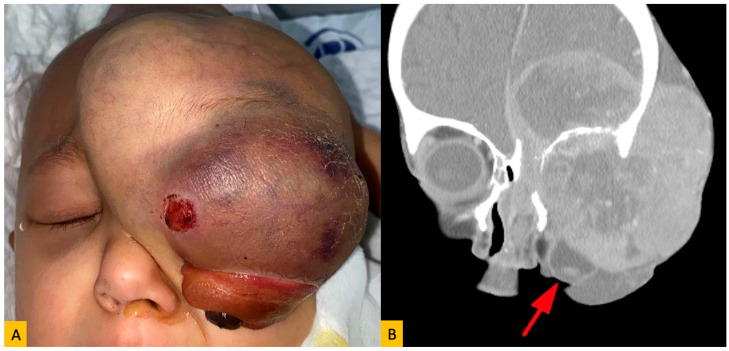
(A) Clinical photograph of a 4-year-old child with orbital metastasis of neuroblastoma showed proptosis, eyelid swelling and chemosis of the left eye. (B) Coronal computed tomography showing a massive metastatic lesion with intracranial, extracranial and intraorbital extensions in the left orbital roof, causing a protruded appearance in the left globe (red arrow) and displacing inferiorly.
